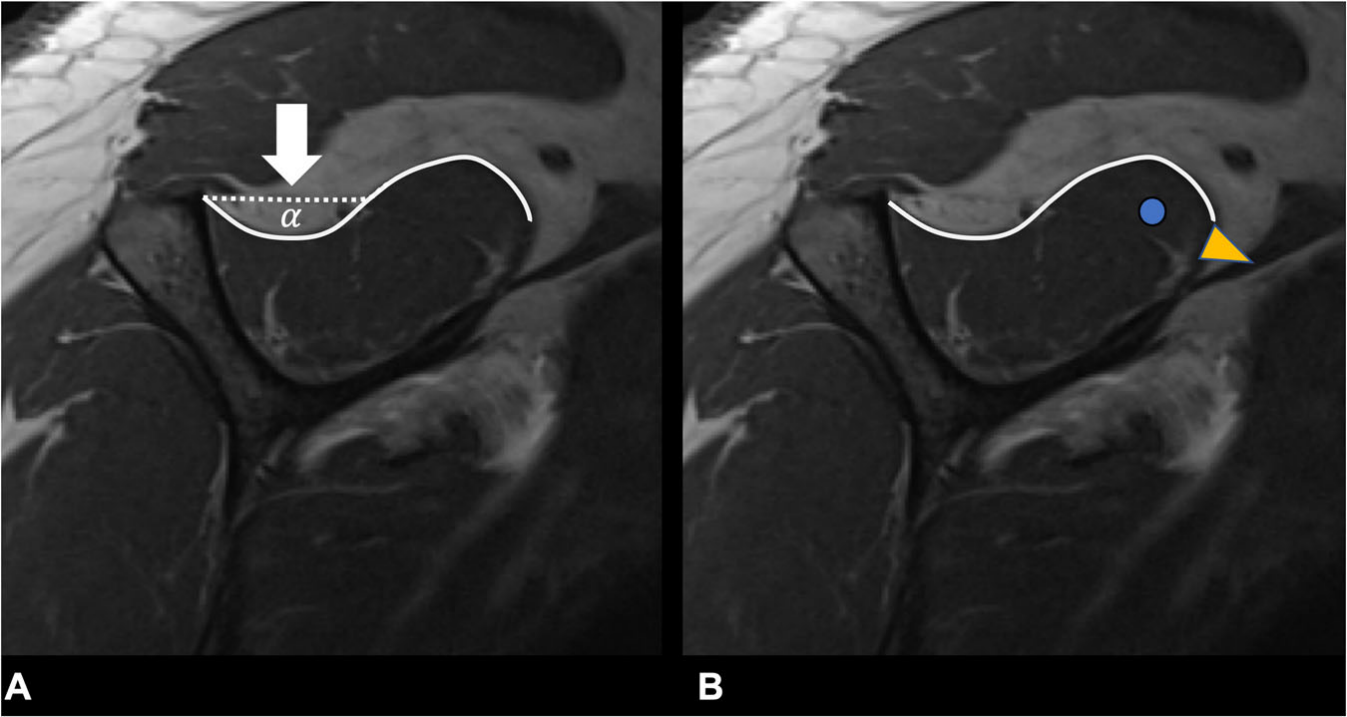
Sagittal oblique T1-weighted images of a 59-year-old patient with early SSP muscle atrophy resulting in a positive blackbird sign (A, B). The blackbird sign is assessed at the centre of the SSP muscle. The atrophy of the supero–posterior SSP muscle subregion creates an inwardly curved muscle contour, giving the impression of an anterior-facing blackbird. In contrast, the medial and inferior SSP muscle subregions appear unchanged, while the atrophied region is filled with fatty tissue (asterisk)
